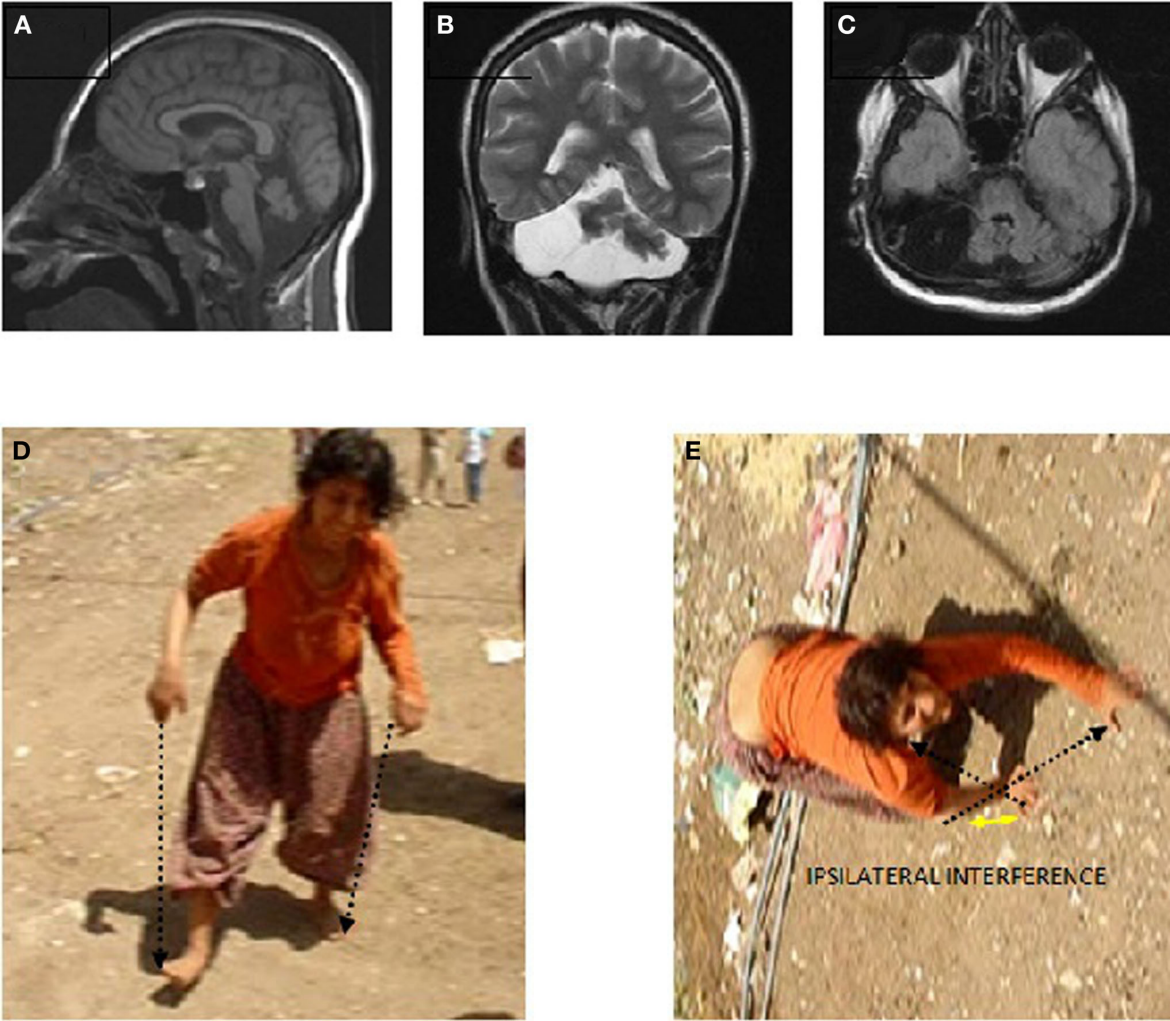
Case IV-23. Sagittal (A), coronal (B), and axial (C) MRI scans. (D) Parallel-sequence ataxic BL: right arm-right leg and left arm-left leg (see arrows); (E) diagonal-sequence QL: left arm-right leg and right arm-left leg (see arrows).
